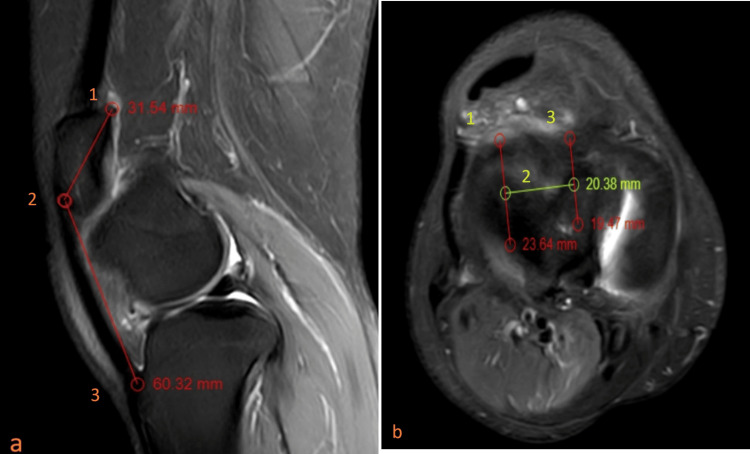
MRI of the right knee (a) Proton density fat saturation sequence in the sagittal section showing an Insall-Salvati ratio > 1.5, where points 1 to 2 represent the length of the patella bone and points 2 to 3 represent the length of the patella tendon. (b) Proton density fat saturation sequence in the axial section showing a tibial tuberosity to trochlear groove distance of 20.38 mm, where point 1 represents the trochlear groove, point 3 represents the tibial tuberosity, and point 2 represents the distance between the tibial tuberosity and trochlear groove
